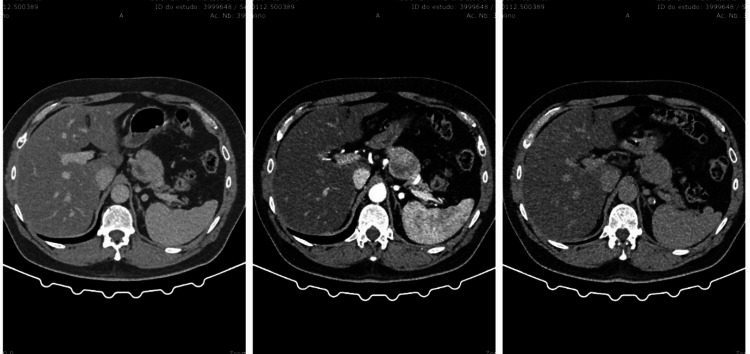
Multiple phase CT scan abdomen and pelvis with contrast (portal-venous, early-arterial, late-arterial phase). Lesion noted to the body of the pancreas.
